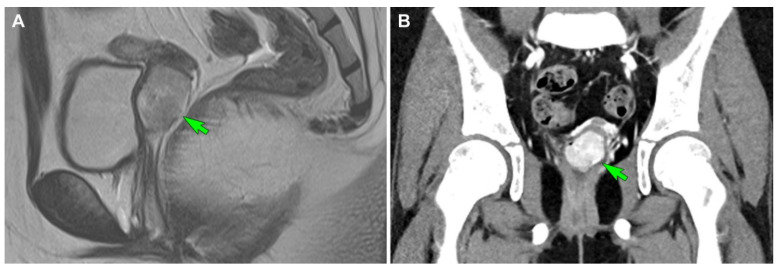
Imaging findings (case 2). (A) Initial T2-weighted turbo spin-echo sagittal magnetic resonance imaging reveals a well-circumscribed hypervascular mass (green arrow) originating in the uterine cervix, with heterogeneous high signal intensity and a lobulated contour. (B) Initial contrast-enhanced computed tomography shows an enhancing mass (green arrow) abutting the uterine cervix.
